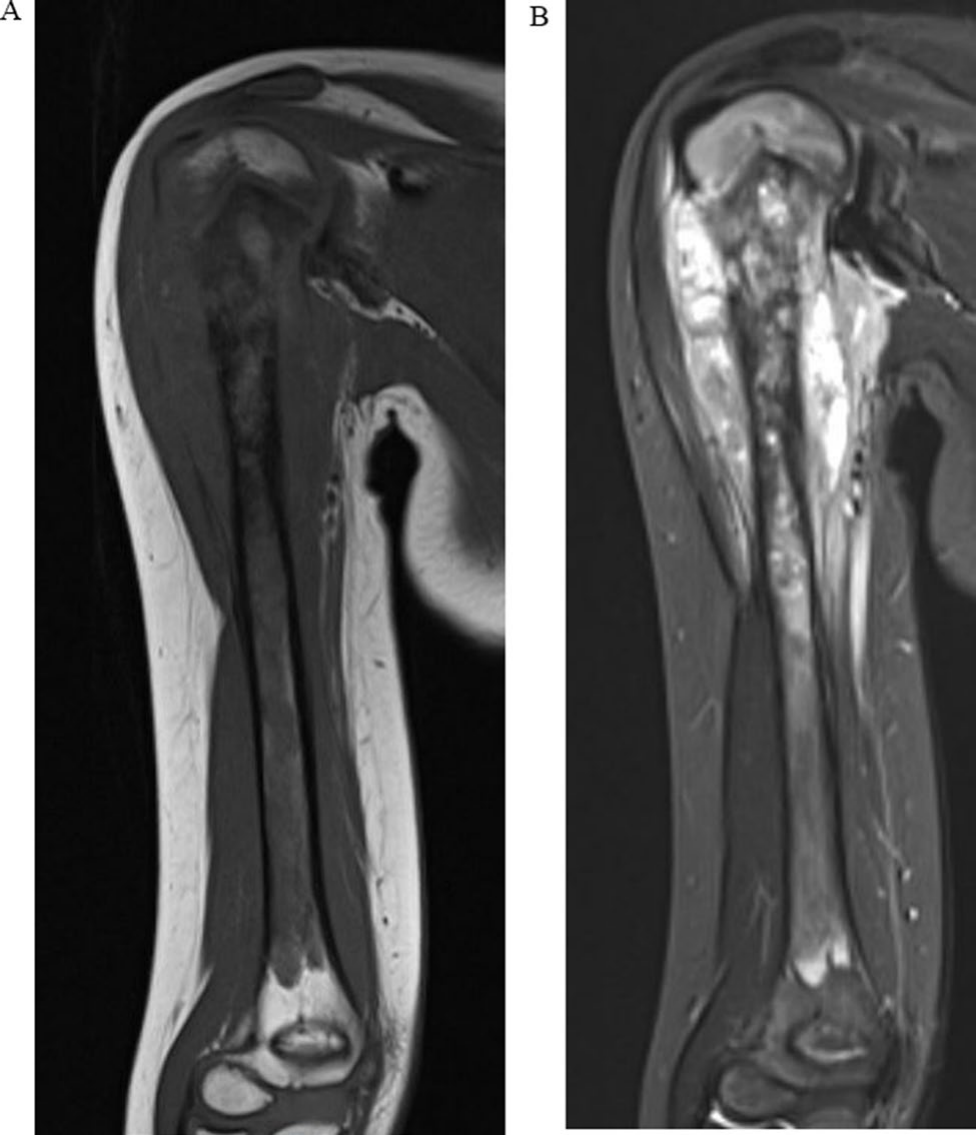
Preoperative tumor extension of a high-grade osteosarcoma of the right humerus (patient #6; MRI: (a) coronar T1 TSE, (b) coronar TIRM)
